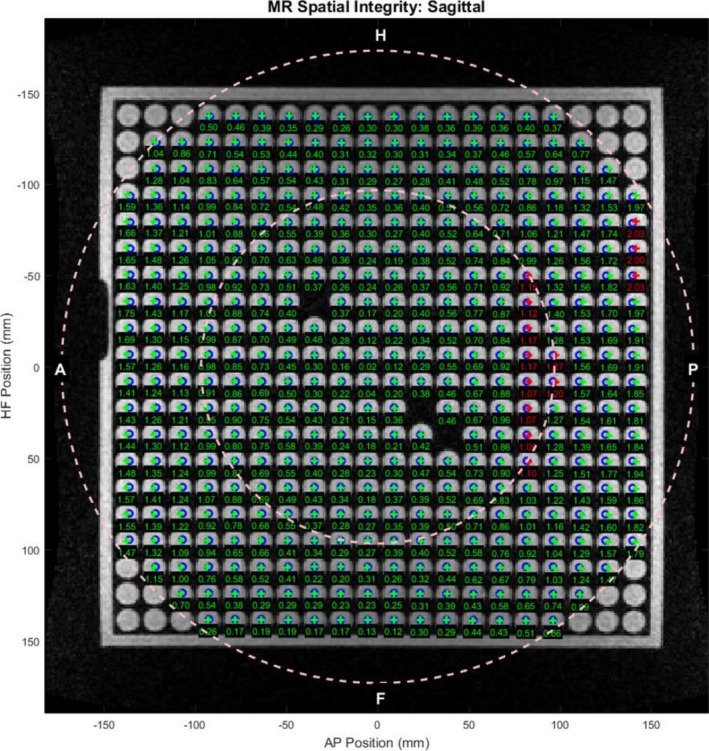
Software analysis results for sagittal 3D TrueFISP slice at x = 0 (TE/TR: 1/3 ms, 60^0^, 1.5 × 1.5 × 3 mm, 534 Hz/pixel, 81 s) on the MRI‐Linac using standard shimming at gantry angle 0^0^. Green indicates a passing score for the location while red is failing. The failed test was caused by improper calibration of the Y‐gradient. MRI, magnetic resonance imaging.
